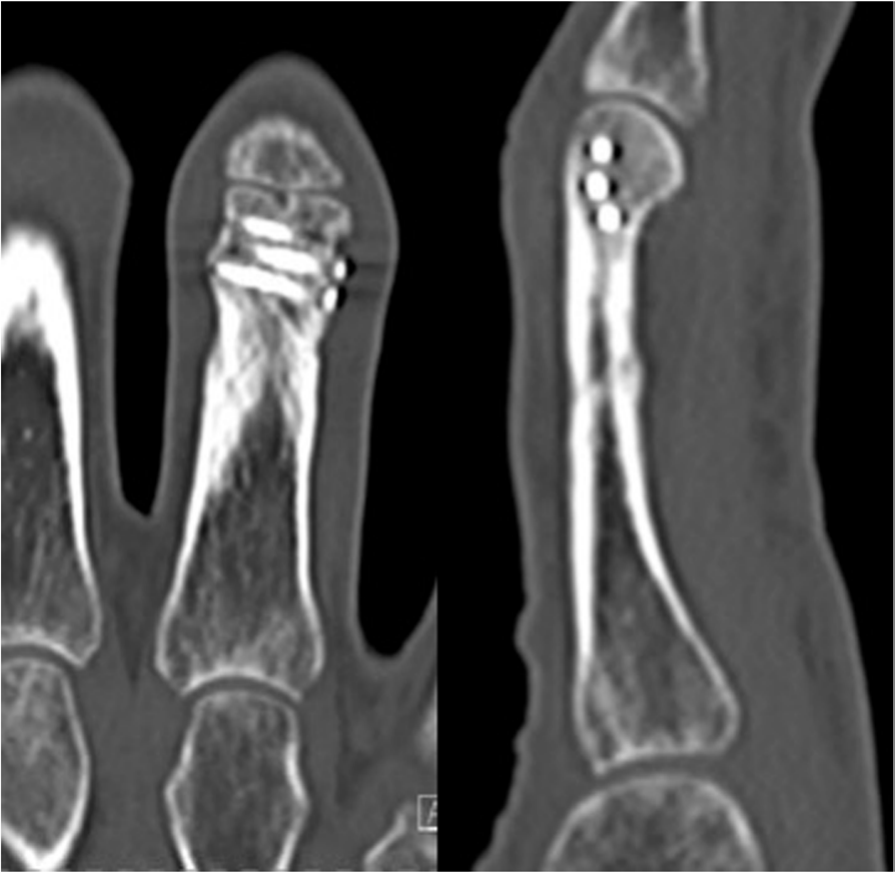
CT scans two months postoperatively showing joint congruency and consolidation
